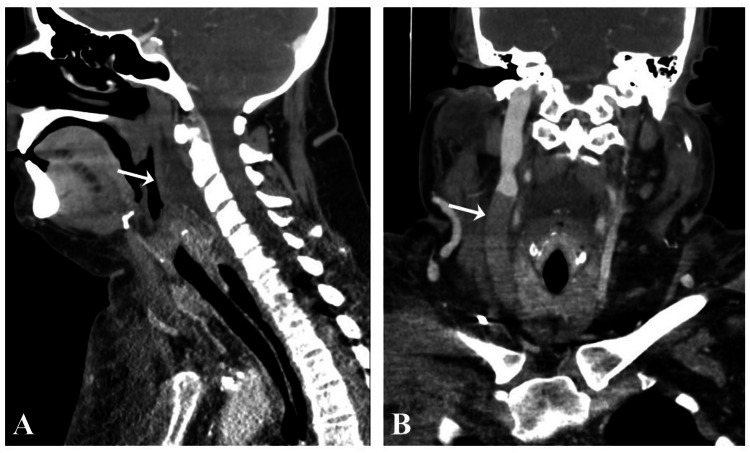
Contrast-enhanced CT scan of the neck revealing (A) retropharyngeal soft tissue edema and/or effusion with retropharyngeal/prevertebral soft tissue thickening (mild generalized subcutaneous soft tissue edema of the visualized bilateral anterior chest wall, neck, and submandibular regions) and (B) diffuse hypo-enhancing appearance of the lower portion of the right internal jugular vein. CT: computed tomography
